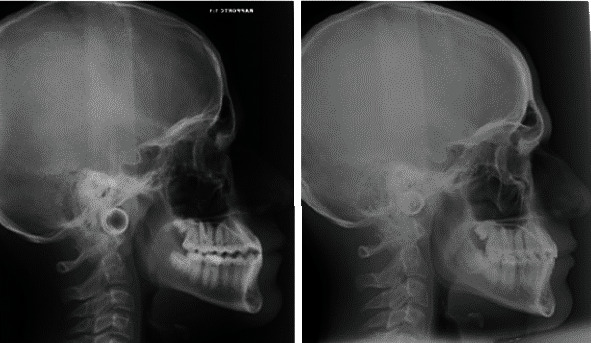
Pretreatment and posttreatment radiographs compared.
